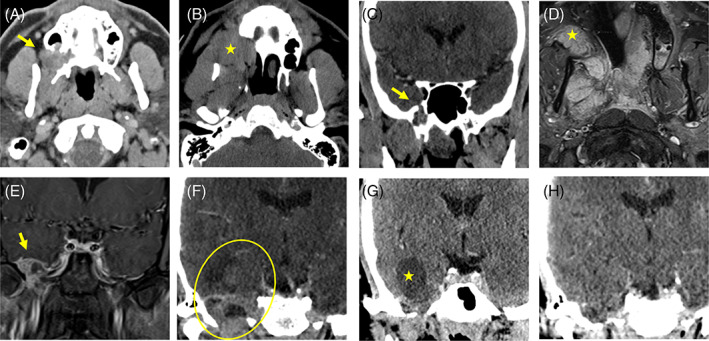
Radiologic findings throughout the disease course. *First recurrence (2013)*: Axial contrast‐enhanced CT (A) demonstrates part solid and cystic mass (arrow) centered in the right first maxillary molar alveolus with bony erosion and extension into the maxillary sinus. *Second recurrence (2016)*: Axial contrast‐enhanced CT (B) shows recurrent mass with increased involvement of the right hard palate and extension into right pterygopalatine fossa and masticator space. *Third recurrence (2018)*: Coronal contrast‐enhanced CT images (C) show increased infratemporal fossa involvement with expansion of right foramen rotundum and suspected early intracranial extension into middle cranial fossa (arrow). *Pre‐pembrolizumab baseline (2019)*: Axial (D) and coronal (E) contrast‐enhanced T1 weighted magnetic resonance imaging now 1.5 years after skull base resection, right total maxillectomy, and septoplasty shows extensive multifocal recurrence with expansile, enhancing masses in the right middle cranial fossa (arrow), masticator space, and submucosal buccal space (star). *Progression on pembrolizumab (2019)*: Off‐coronal contrast‐enhanced CT (F) after four cycles of pembrolizumab demonstrates progression, with enlarging solid and cystic intracranial tumor in the right middle cranial fossa (oval). *Response to erdafitinib (2020)*: Off‐coronal contrast‐enhanced CT 1 month (G) and 4 months (H) after start of erdafitinib show initial decrease in solid component with persistent cyst in the right middle cranial fossa (star), followed by marked decrease in intracranial component of tumor.
